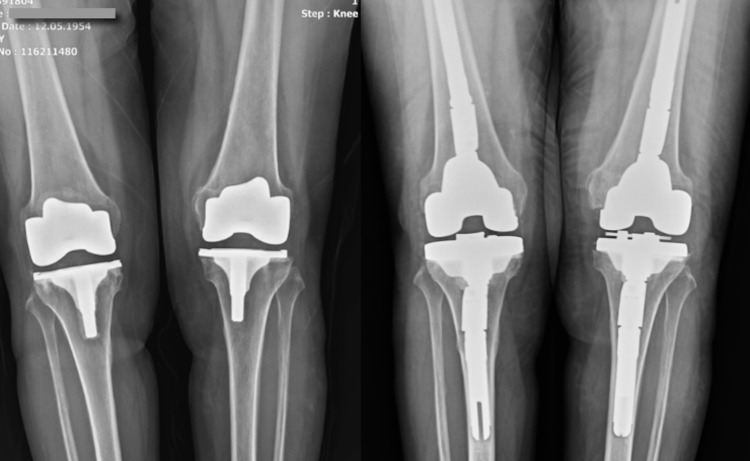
Case 2 Preoperative and Postoperative Knee X-Ray
